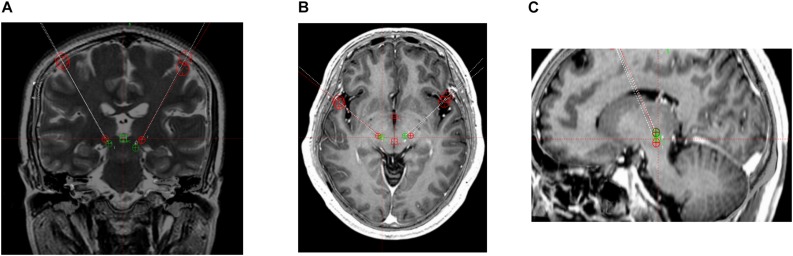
3 T magnetic resonance images. Target coordinates of the posterior subthalamic area (PSA) were 10 mm lateral, 7.7 mm anterior, and 3.4 mm superior. PSA (green) and ventral intermediate nucleus of the thalamus (red) contacts. (A) Coronal T2-weighted image. (B) Horizontal T1-weighted image. (C) Sagittal T1-weighted image.
